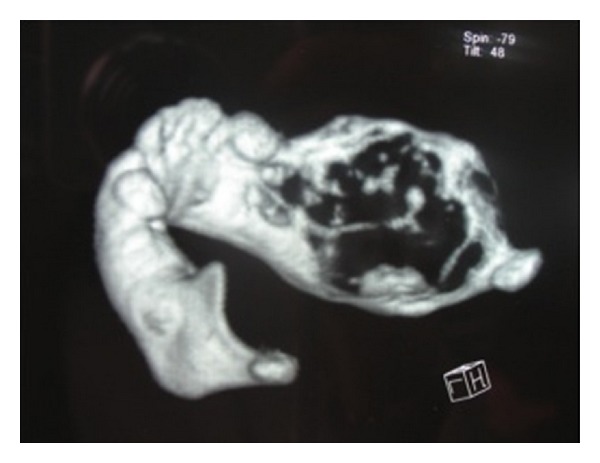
Computed tomography of the mandible showing the extension of the tumor area.
